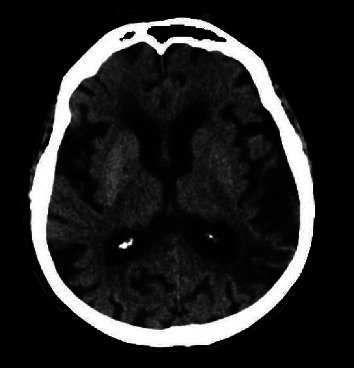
Patient's noncontrast brain computed tomography.
